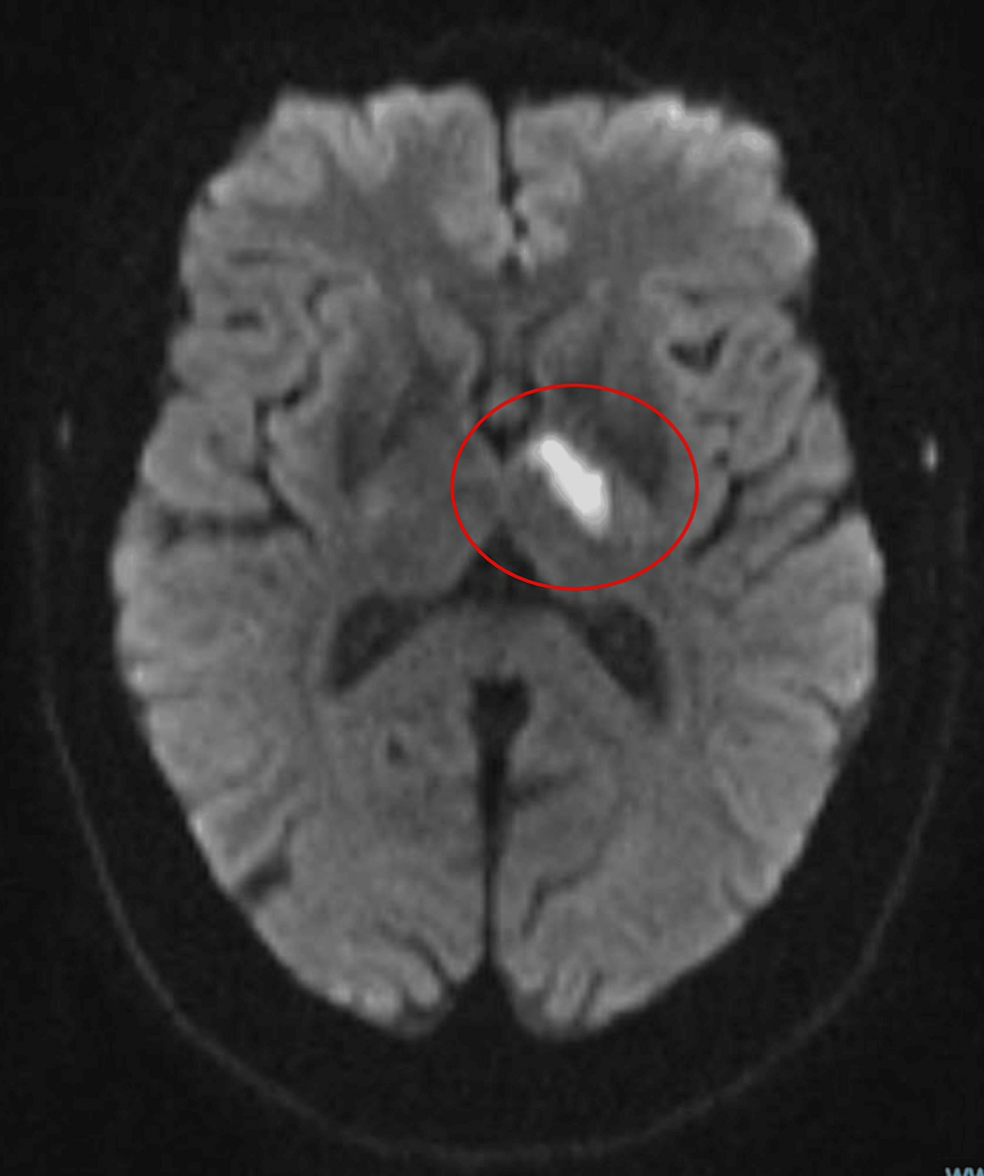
MRI brain confirming acute left basal ganglia ischaemic stroke
